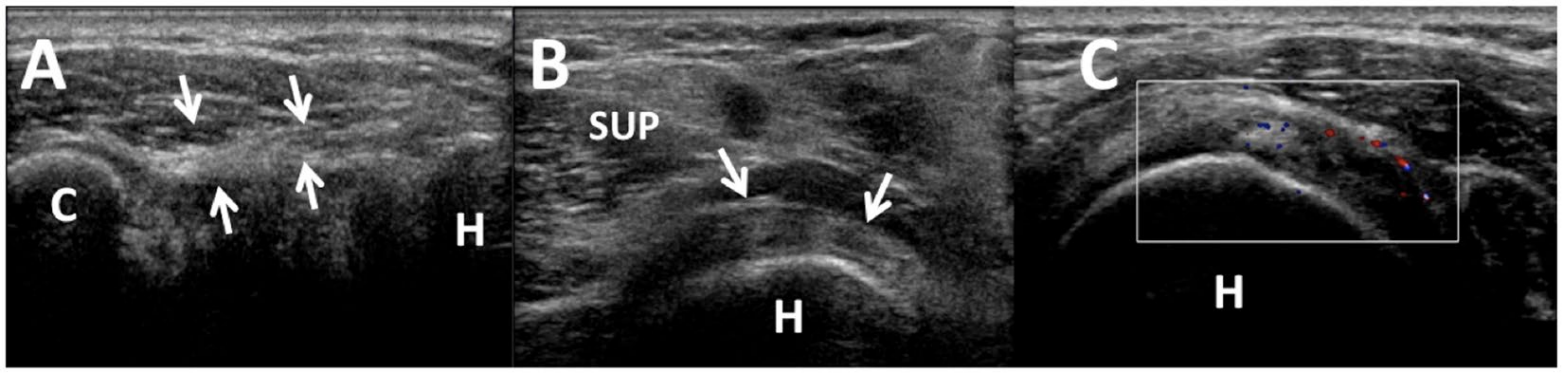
US findings of frozen shoulder patients: (A) Gray-scale US showed a thickened CHL (arrowheads); (B) Gray-scale US showed a thickened inferior capsule; (C) Color Doppler detected an increased vascularity and echotexture in the rotatol cuff interval; C, coracoid; SUP, suparaspinatus tendon; H, humeral head.
